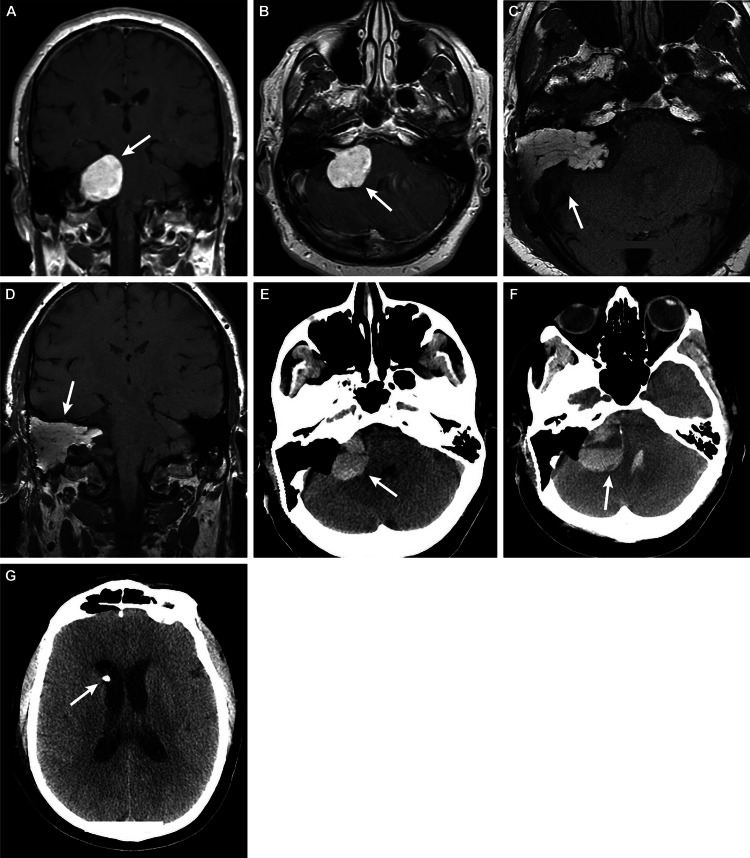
Case 4 – a 56-year-old man who presented with right-sided hearing loss Preoperative (A) coronal and (B) axial T1-weighted MRI indicated a right vestibular schwannoma (arrows) measuring 3.7 × 3.8 × 3.3 cm, causing brainstem compression and mass effect on the right cerebellum. Postoperative (C) axial and (D) coronal T1-weighted MRI demonstrated expected postoperative changes related to a right translabyrinthine craniotomy for resection of the large vestibular schwannoma, with fat-packing (arrows) in the mastoid air cells. Mass effect on the right brainstem and cerebellum was not significantly changed. (E) Axial CT on postoperative day nine showed postoperative changes and a new 3.4 × 2-cm hyperdensity (arrow) consistent with a hemorrhage in the right cerebellopontine (CP) angle operative bed. It indicated an increased mass effect on the right middle cerebellar peduncle and pons and on the fourth ventricle. (F) Axial CT performed on postoperative day 10 showed evidence of an expanding hematoma (arrow), now 4 × 3 cm, causing further increased mass effect. Blood products could also be viewed in the fourth ventricle. (G) Axial CT showed the placement of an external ventricular drain (arrow) in the frontal horn of the right lateral ventricle following the surgical evacuation of the hematoma MRI: magnetic resonance imaging; CT: computed tomography Used with permission from Barrow Neurological Institute, Phoenix, Arizona
